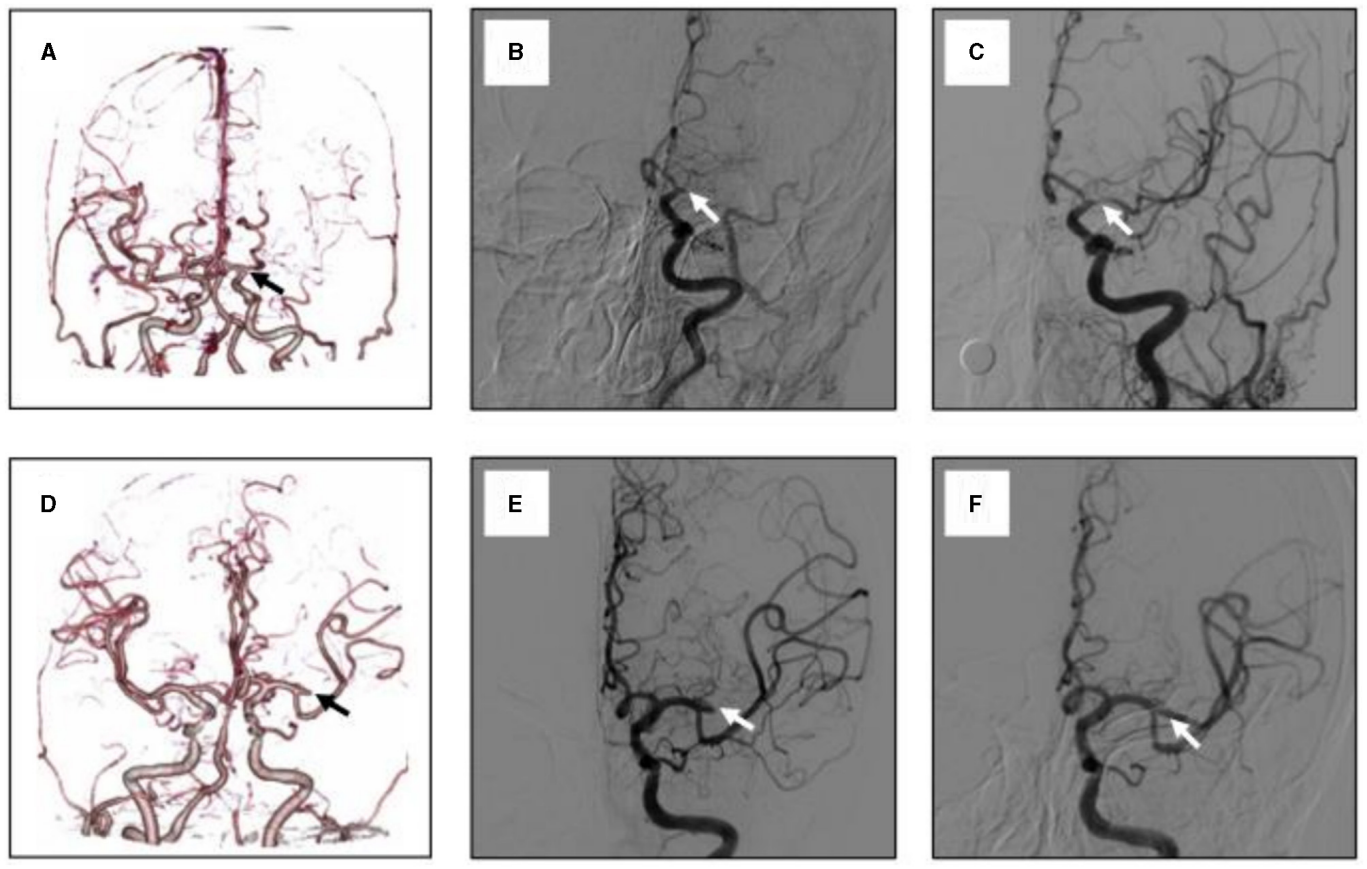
Representative cases of clot pattern at the occlusion site of MCA as visualized by CTA and DSA. (A) A maximum intension projection reconstruction image of CTA that showed left MCA occlusion, with the clot pattern being tapered sign. (B) The initial angiogram confirmed the same cite of occlusion. (C) The recanalization of the occluded MCA after stent retriever, the occluded MCA are recanalized, but the angiogram showed a focal stenosis of more than 50% at the M1 trunk. (D–F) shows another patient with left MCA occlusion. (D) with clot pattern being cut-off sign. (E) The initial angiogram also confirmed the same cite of occlusion. (F) Successful recanalization of the occluded MCA without stenosis after SR thrombectomy.
